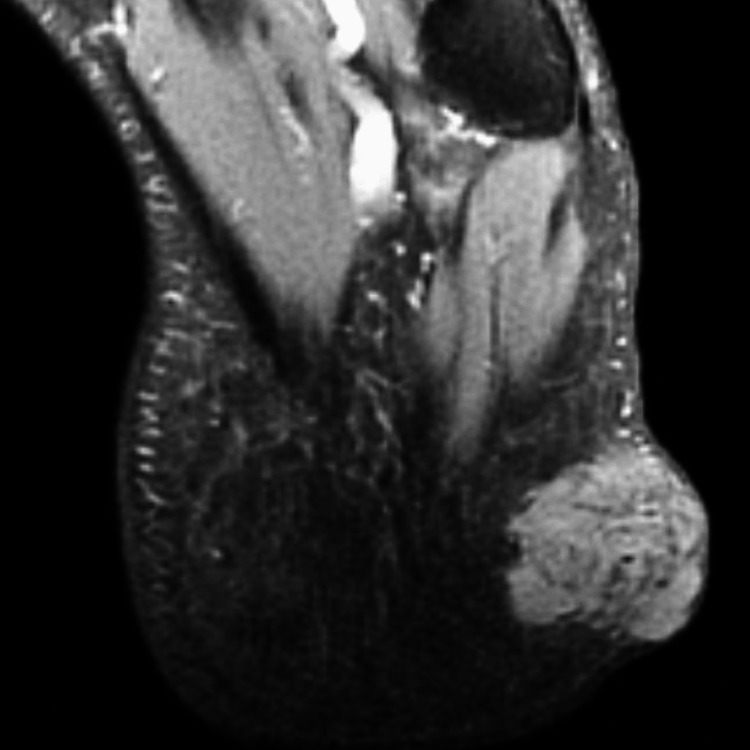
MRI T2 proton density showing subcutaneous well circumscribed tumor.
